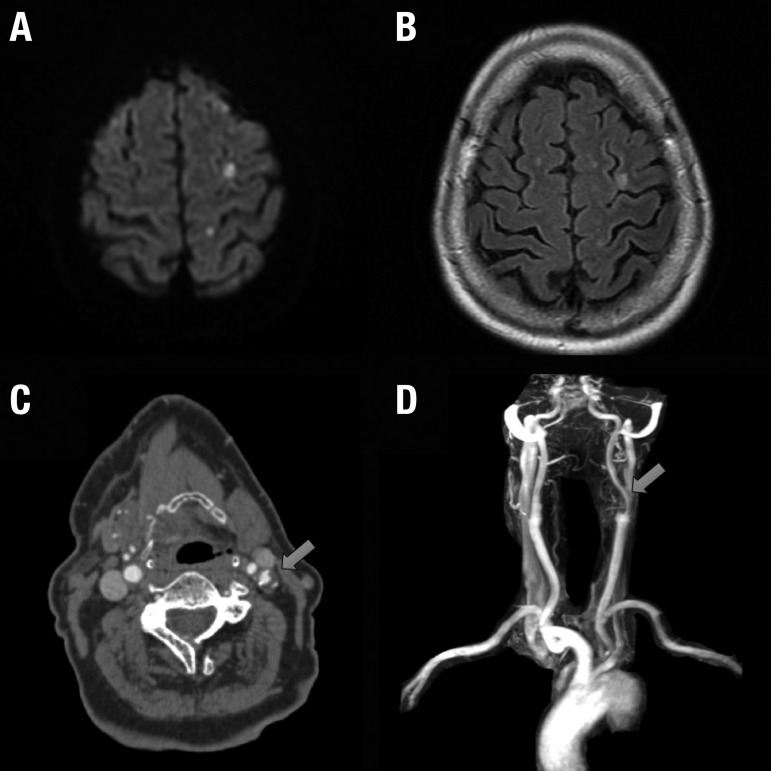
Diffusion-weighted imaging (A) and T2-FLAIR (B) MRI showing multiple small hyperintense foci in the middle frontal and post central gyri. Computer Tomography Angiography (C) and MR Angiography (D) showing mild atheromatosis in both carotid arteries. The left atherosclerotic plaque is soft and ulcerated, causing vessel stenosis of approximately 50%.
